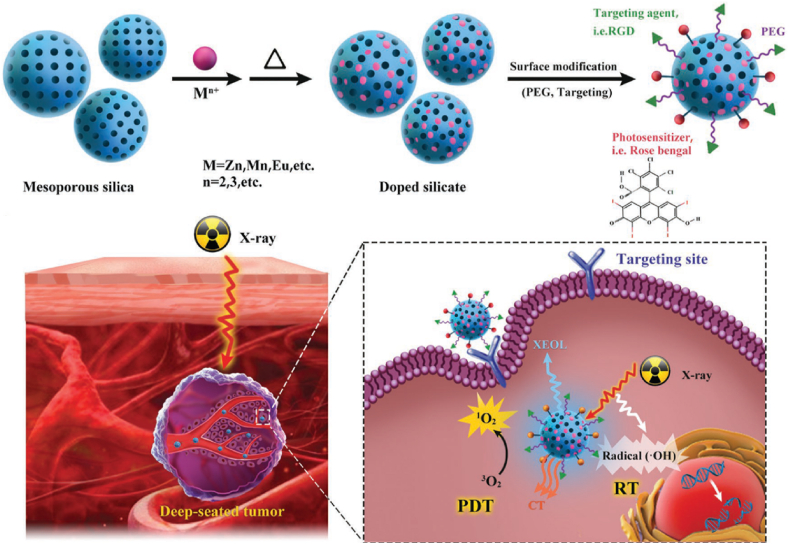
X-ray-induced deep-tissue-penetrating photodynamic therapy using mesoporous silicate nanosensitizers. Adapted from Ref. [44]. Copyright 2017 WILEY-VCH.
